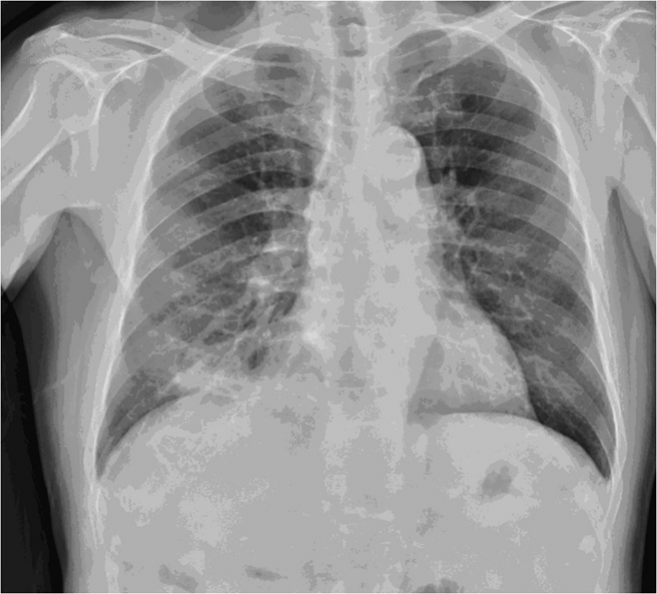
Shadows are noted in the lower right region of the lungs on a chest X-ray.
